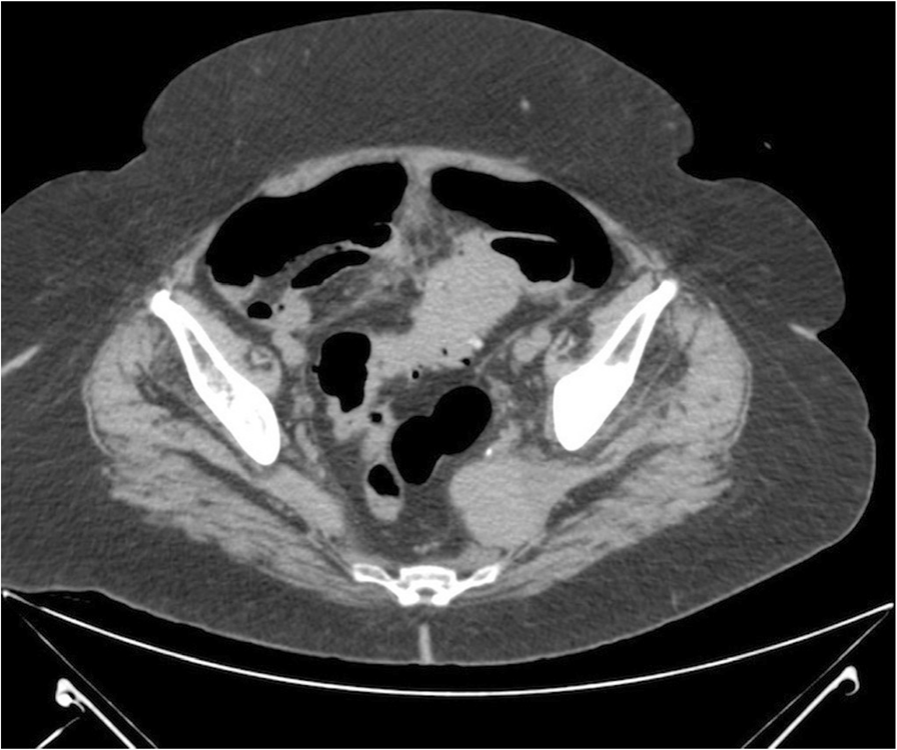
Axial computed tomography with no enhancement depicts a mass of soft tissue density in the anatomical area of the sigmoid colon and another compact lesion of soft tissue density on the left lateral wall of the minor pelvis. Presence of diverticula can be seen
